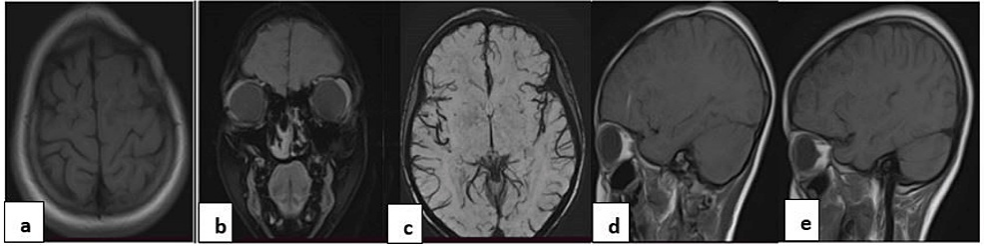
MRI of the head, axial unenhanced T1W image, showing subtle effacement of the sulci of the left frontal lobe and thinning of the ipsilateral subcutaneous scalp tissues (a), coronal T1W image post gadolinium enhancement showing a marked reduction in the left nasal cavity and subtle effacement of the sulci (b), axial SWI revealed no micro hemorrhages or aneurysms (c), the left para sagittal MRI (d) also showed thinning of the subcutaneous frontal scalp tissues and subtle frontal lobe atrophy compared with the contralateral right parasagittal image (e). MRI, magnetic resonance imaging; T1W, T1-weighted; SWI, susceptibility-weighted imaging.
